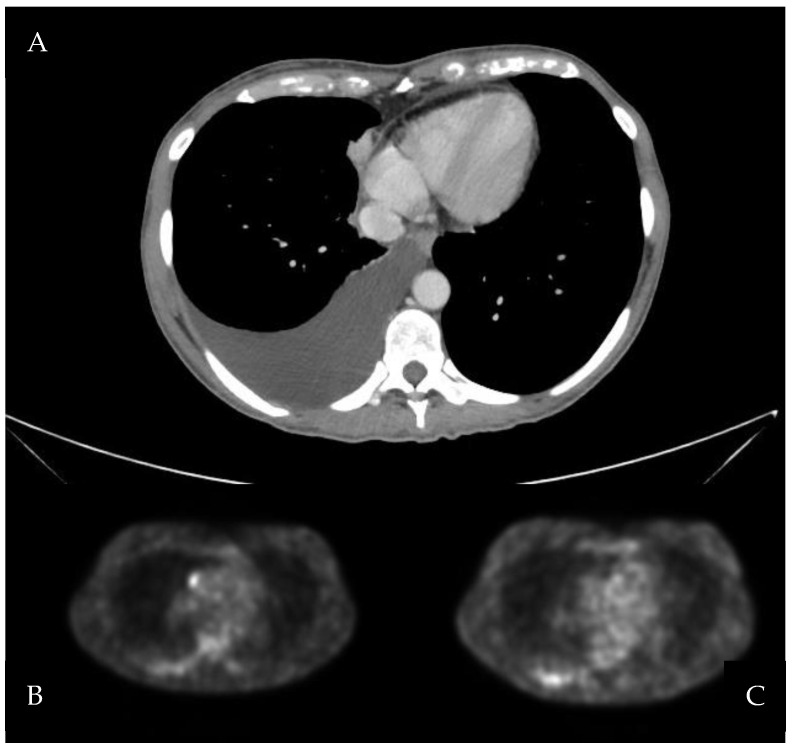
(A): axial section of CT scan showing right pleural effusion and ipsilateral nodular irregularities of parietal and mediastinal pleura. (B,C): axial sections of ^18^F-FDG-PET scan showing increased metabolic activity of right pleural nodules.
